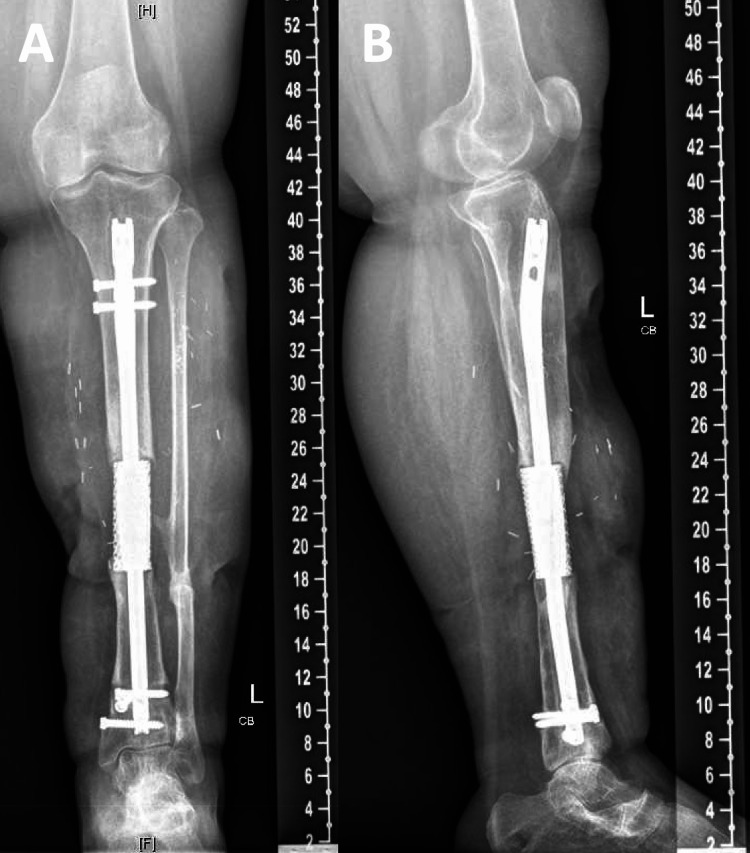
One-year postoperative (A) anteroposterior and (B) lateral radiographs of the left tibia demonstrating 3D-printed titanium implant and intramedullary nail with restored alignment and segmental bony defect. 3D: three-dimensional
